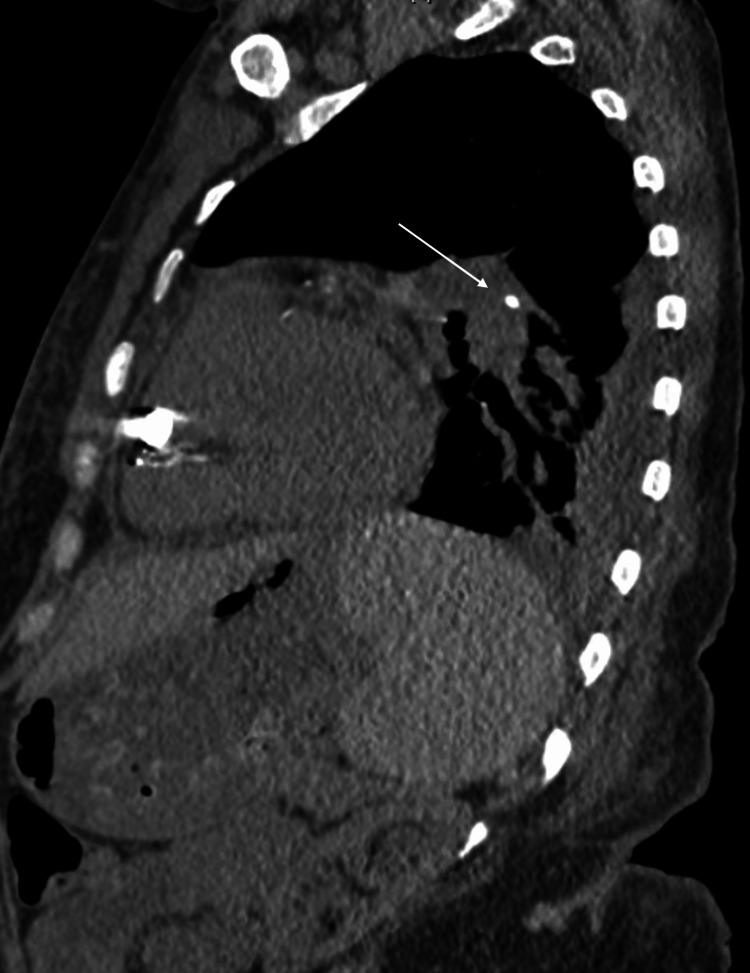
Non-contrast sagittal chest CT demonstrates calcific embolism within the left pulmonary artery.
